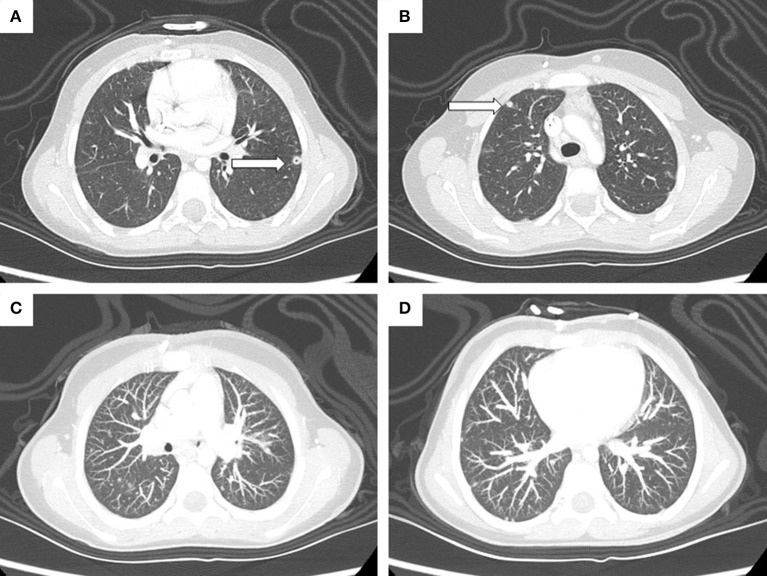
High-resolution CT scan highlights a 6-mm cavitated nodule (arrow) in the anteromedial segment of the left inferior lobe (A) and a 5-mm subpleural nodule (arrow) in the anterior segment of the right superior lobe (B). Maximum intensity projection (MIP) reconstruction image (C,D) shows the presence of multiple disseminated parenchymal and subpleural nodules (max. 5 mm).
